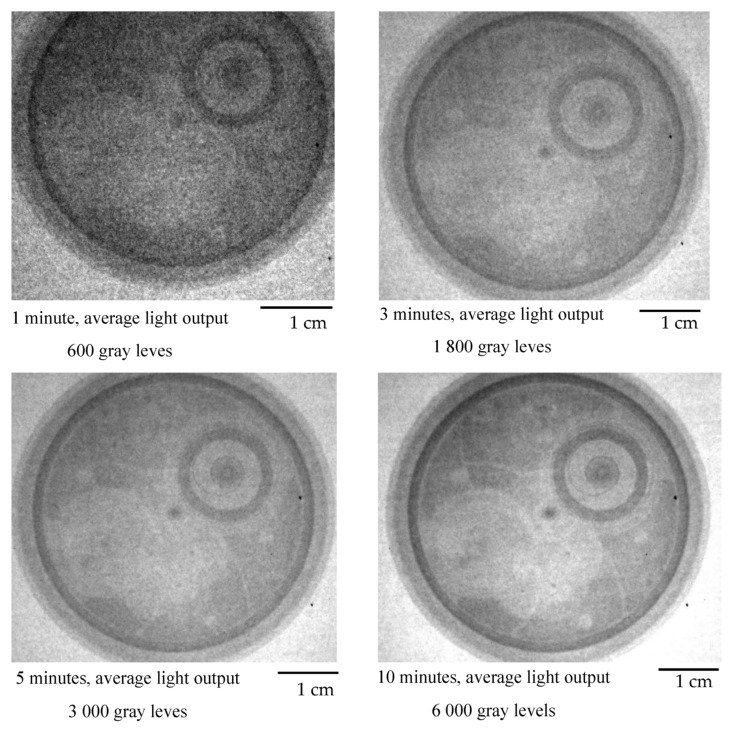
Neutron radiograph of a pocket watch at different exposure times (L/D = 20). Grayscales are stretched for optimal display.
